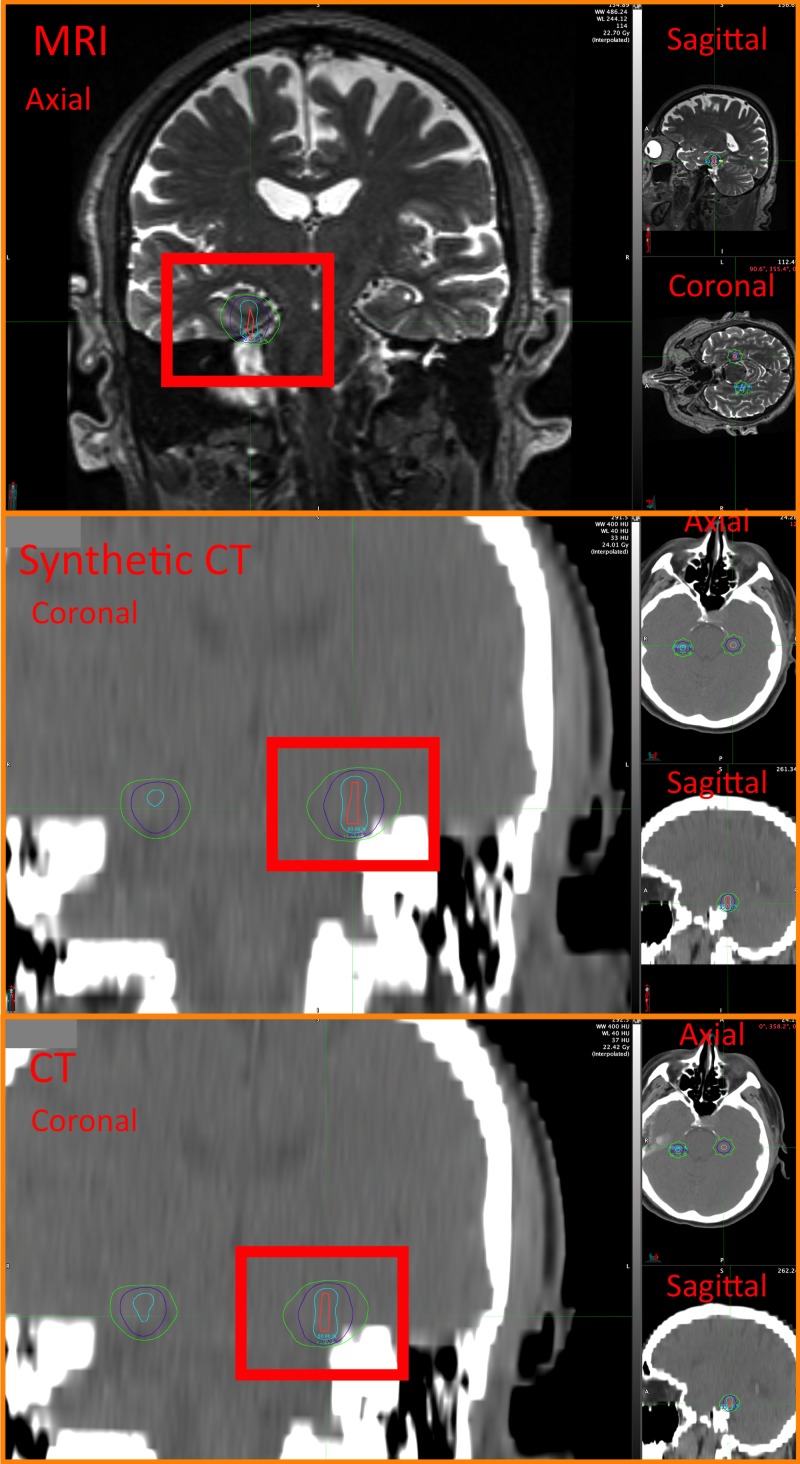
Representation of SRS plans for single metastasis brain tumor (orange color) at multiple planes (axial, sagittal, and coronal) in MRI images (top) using TMR (10) algorithm and synthetic CT (middle) and CT (bottom) using convolution algorithm. The 50% isodose line (cyan), 20% isodose line (purple) and 12% isodose line (green) has been demonstrated for all images and plans. Gy = Gray; TMR = Tissue Max Ratio; CT = Computed Tomography; MRI = Magnetic Resonance Imaging ; SRS = Stereotactic Radiosurgury
